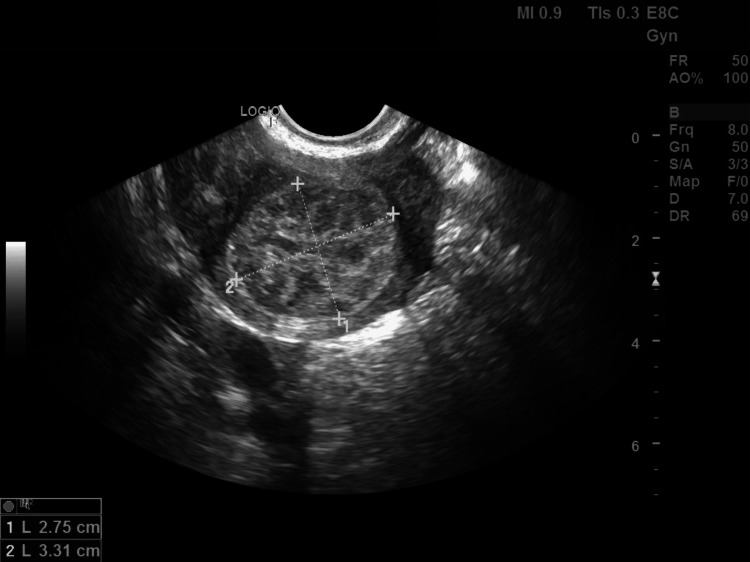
A transvaginal ultrasound of the pregnancy remnants measuring 2.75cm x 3.31cm once beta-hCG levels returned to undetectable levels. This image was obtained six months after administration of methotrexate for medical termination of pregnancy in the rudimentary uterine horn.
